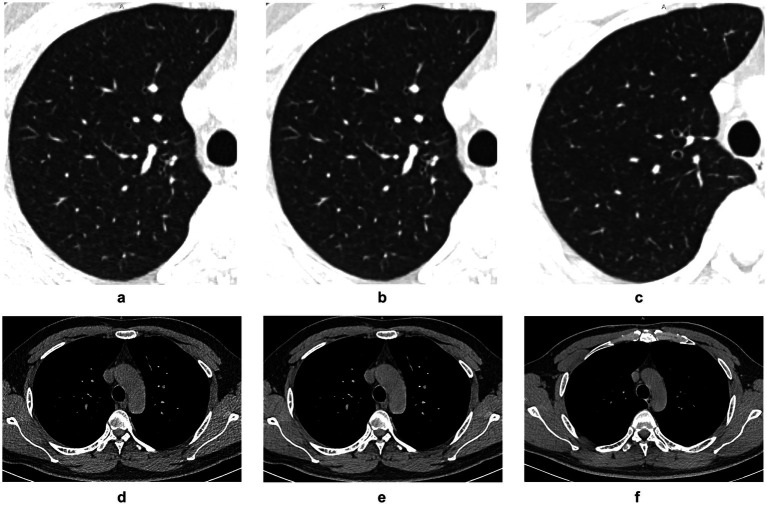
Representative axial unenhanced chest CT images from two participants with comparable body habitus. (a–c) Lung-window images: LD-SAFIRE (a), LD-AiR (b), and SD-SAFIRE (c). (d–f) Mediastinal-window images: LD-SAFIRE (d), LD-AiR (e), and SD-SAFIRE (f). Panels a, b, d, and e were from the same low-dose participant, whereas c and f were from another participant scanned with the standard-dose protocol. LD-AiR shows reduced image noise and improved delineation of pulmonary vessels and mediastinal soft-tissue interfaces compared with LD-SAFIRE, with an overall appearance similar to SD-SAFIRE.
